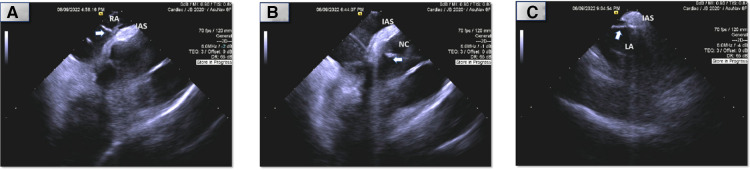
Intracardiac (ICE) echocardiography of ablation sites (arrow: ablation catheter, RA, right atrium; NC, non-coronary sinus of Valsalva; LA; atrial septum; IAS, interatrial septum). *Images are placeholders for videos which are attached. (A) Right atrial septum. (B) Non-coronary sinus of Valsalva. (C) Left atrial septum.
